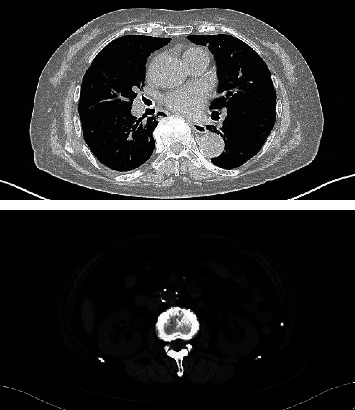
Plain CT of the chest and abdomen showing no findings suggestive of pneumonia, and no abnormality in renal morphology.
